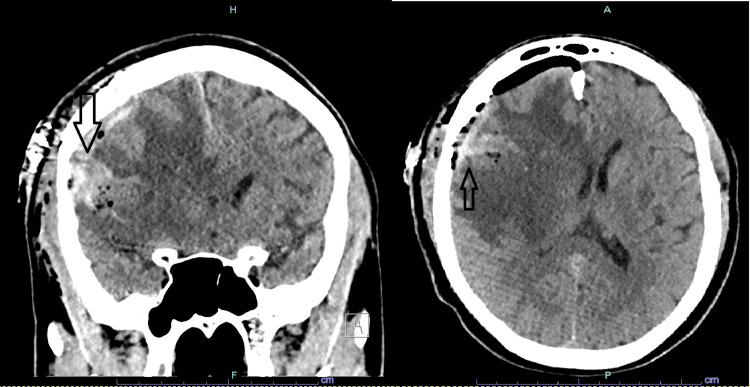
The post-craniotomy CT of the head shows a meningeal origin of the mass in both the coronal and transverse sections.
